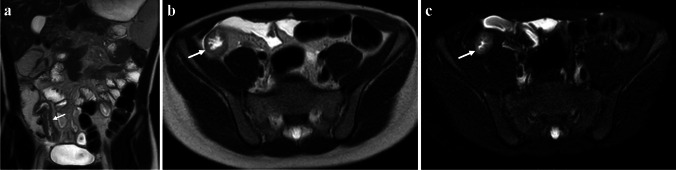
An 11-year-old male with ileal Crohn’s disease (*arrows*). a Coronal T2-weighted single-shot fast spin-echo (SSFSE), (b) axial T2-weighted SSFSE, and (c) axial T2-weighted SSFSE with fat saturation MR images show bowel wall thickening, intramural edema, and radiologic ulcers, yielding an simplified Magnetic Resonance Index of Activity (sMaRIA) score of 4/5. There is no peri-enteric edema
